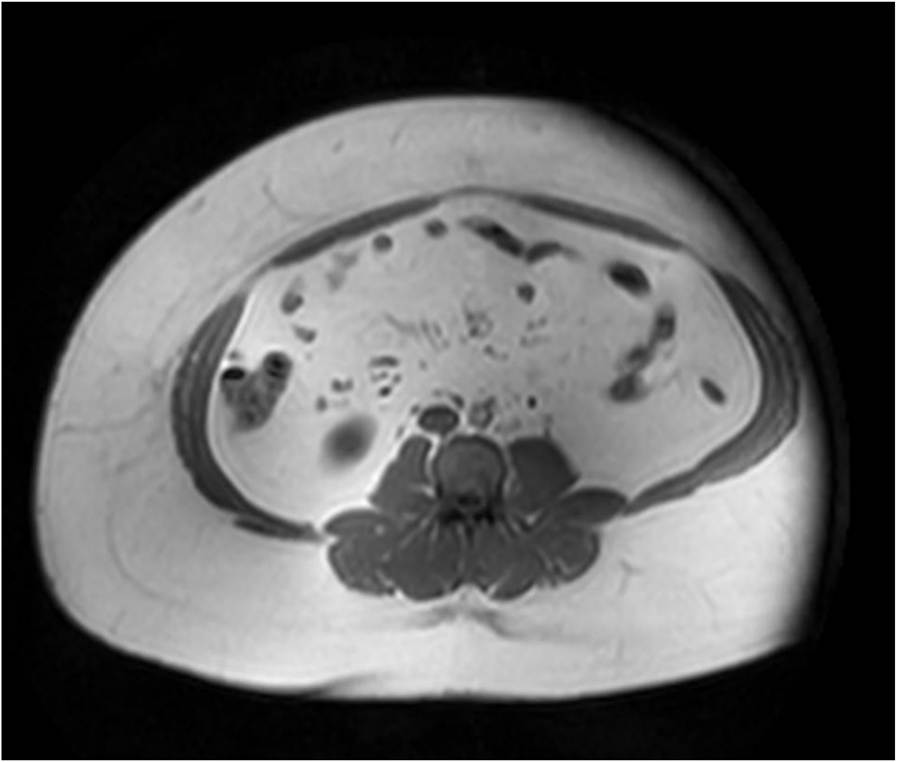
Suggested workaround for adipose tissue quantification in patients with higher degrees of obesity. Sample transverse MR image after patient has been positioned non-centrally (laterally) on the MR table. Full-body fat amounts can be estimated from half-body measures (here: right) using reference/ conversion parameters derived here. MRI acquisition with (obese) patient in central (normal) position is prone to image artifacts or (anatomical) cutoffs on both sides which would prevent proper prediction
